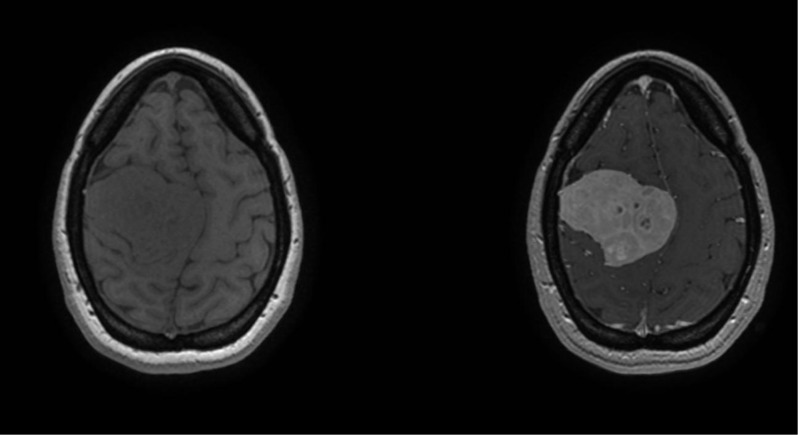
T1-weighted images before and after intravenous administration of gadolinium contrast show heterogeneous contrast enhancement with less enhancement of the central areas.
